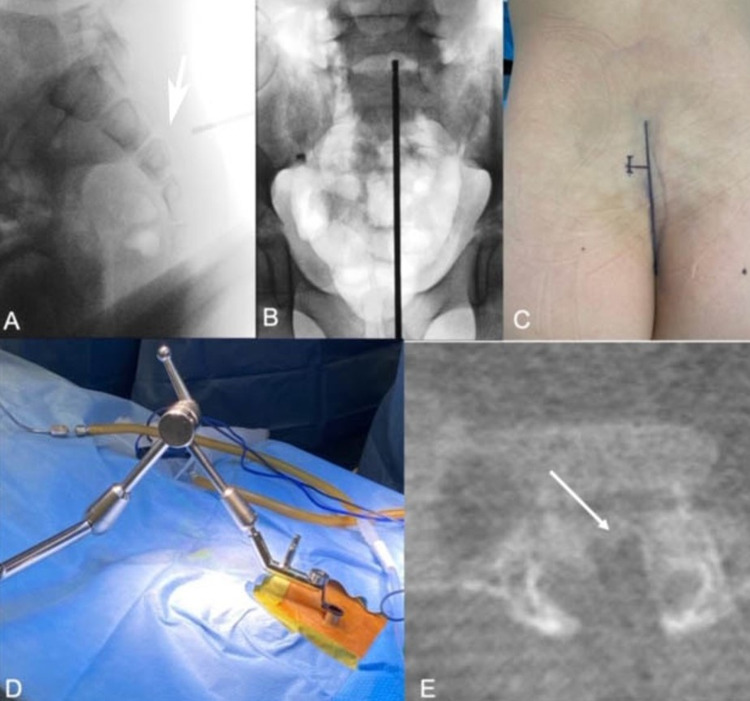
Image showing intraoperative fluoroscopy to localise the S3 level (A) and midline (B) and the final planned 2-cm medial incision (C); placement of dilatation tubes (D); Post-resection CT showing the area of resection (arrow) (E)
